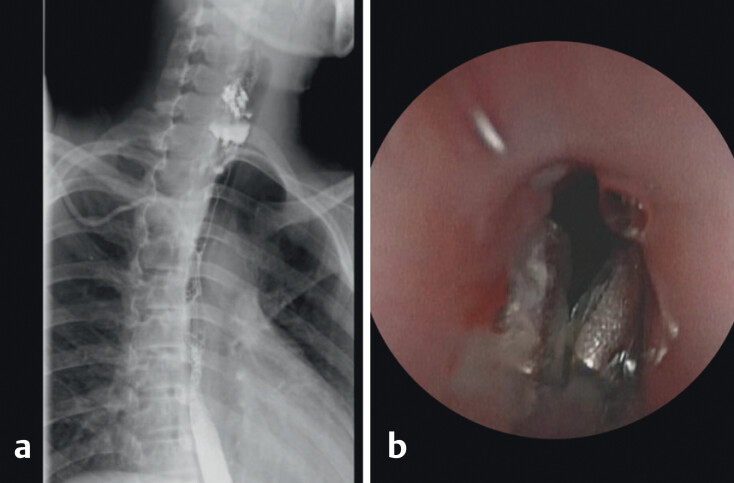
Upper gastrointestinal radiographic and endoscopic images at 2
